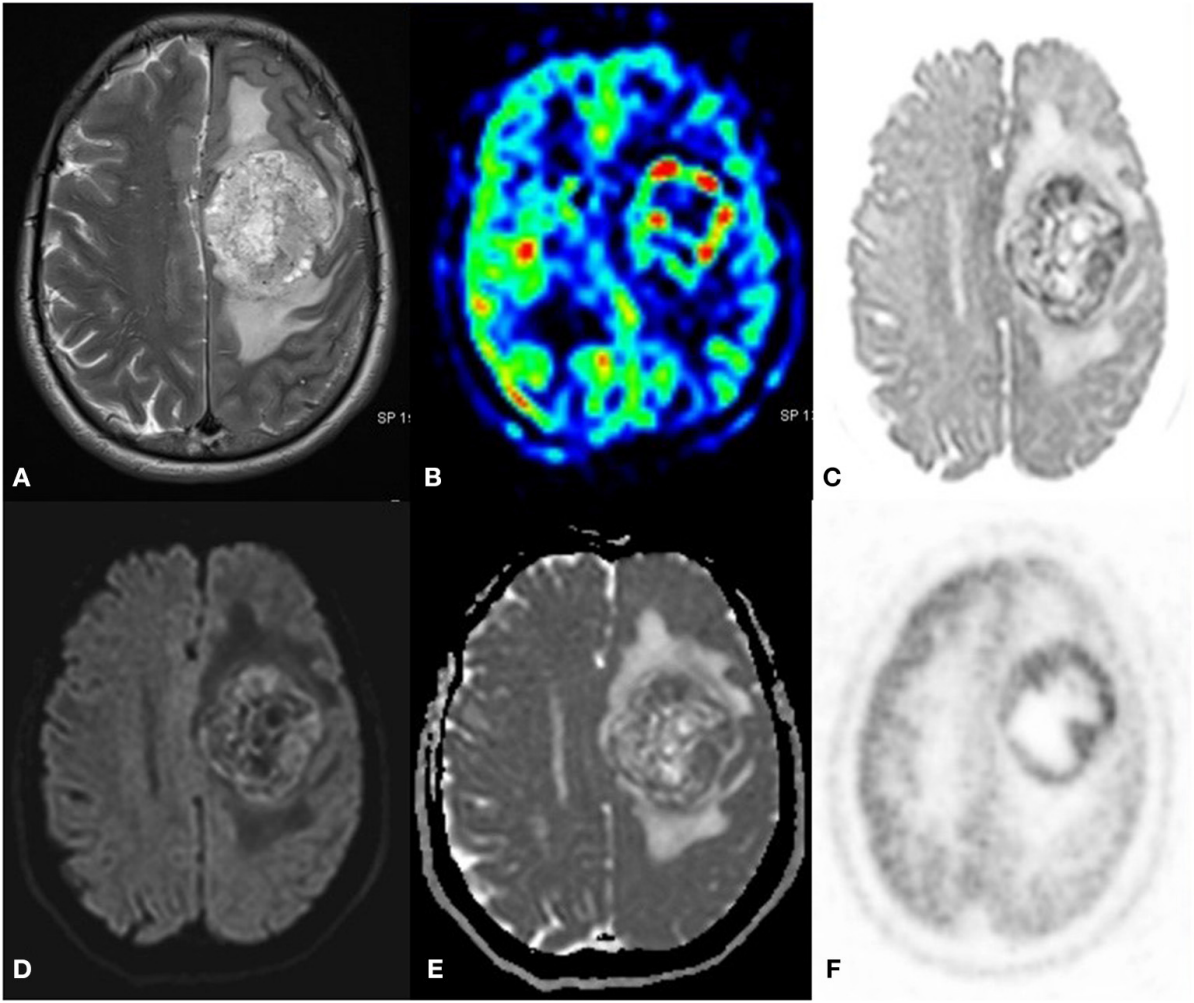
A 55 year old male with a progressive right-side weakness for one and a half month, MRI FDG PET axial images T2 (A) ASL (B) Inverted DWI (C) DWI b2000 (D) ADC (E) NAC PET (F) showed a well-defined lobulated T2 heterogeneous iso- hyperintense with increased perfusion on ASL. The lesion shows DWI restriction with hypermetabolism on PET. There is a match on the inverted DWI image and the NAC PET image. HPE is suggestive of Anaplastic Ependymoma.
